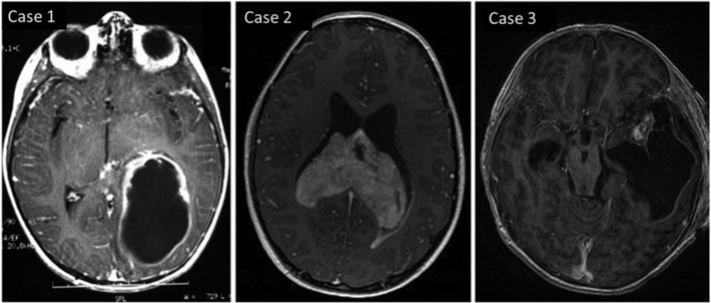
Axial T1-weighted pre-operative MR images for cases 1, 2 and 3 with nodular contrast enhancement and showing a ventricular association
